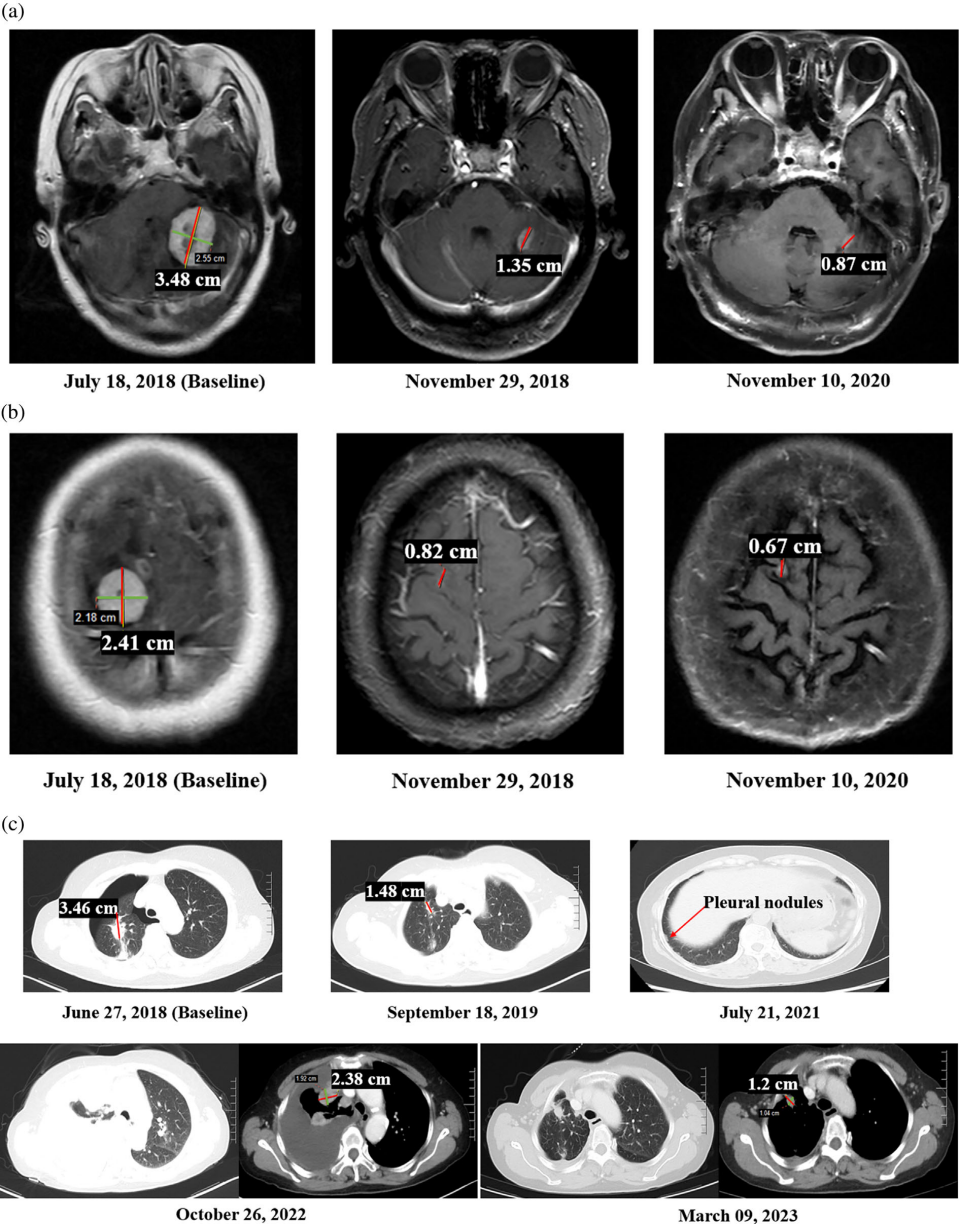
Image screenshots of the three target lesions. (a) Brain magnetic resonance images of the No. 1 target lesion in the left cerebellar hemisphere: it was 34.8 mm in diameter on July 18, 2018 (baseline), 1.35 mm in diameter on November 29, 2018, and 0.87 mm in diameter on November 10, 2020. (b) Brain magnetic resonance images of No. 2 target lesion in the right cerebral hemisphere: it was 24.1 mm in diameter on July 18, 2018 (baseline), 0.82 mm in diameter on November 29, 2018, and 0.67 mm in diameter on November 10, 2020. (c) Thoracic computed tomography scans of No. 3 target lesion in the right upper lung: it was 34.6 mm in diameter on June 27, 2018 (baseline) and 14.8 mm in diameter on September 18, 2019. On July 21, 2021, pleural nodules appeared. On October 26, 2022, the chest CT showed massive pleural effusion and significant progressive disease of the pleural nodules. On March 09, 2023, the pleural effusion disappeared, and the pleural nodules had shrunk significantly.
